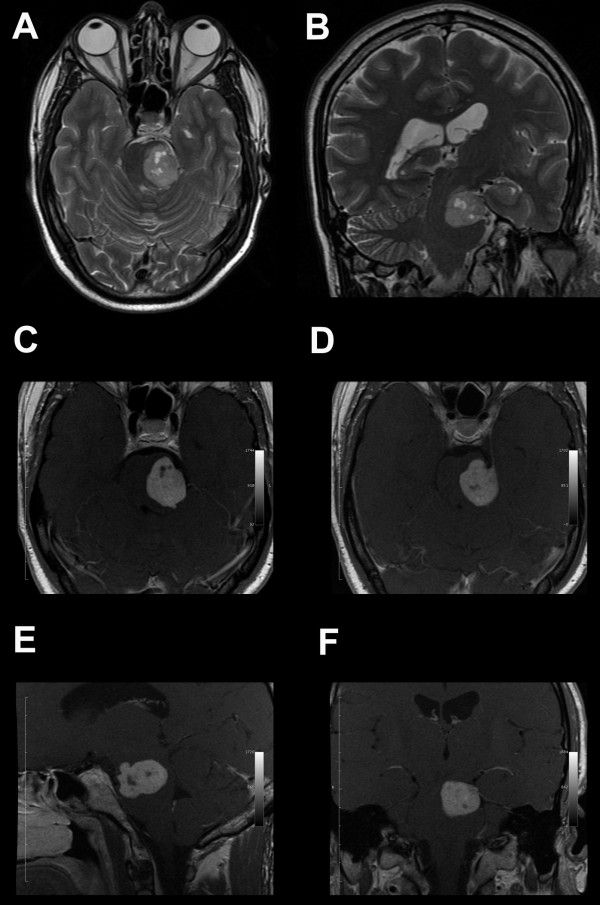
Radiological findings. (A, B). T2-weighted magnetic resonance imaging scan showing a large hyperintense tentorial-based tumor with extension into the pons; microcystic areas are also visible inside the lesion: (A) axial; (B) coronal. (C-F) Post-contrast T1-weighted images demonstrating the gadolinium enhancement of the lesion - the dural tail sign and the tentorial attachment are also shown: (C) axial T1-weighted enhanced image; (D) axial T1-weighted enhanced image; (E) sagittal T1-weighted enhanced image; (F) coronal T1-weighted enhanced image.
